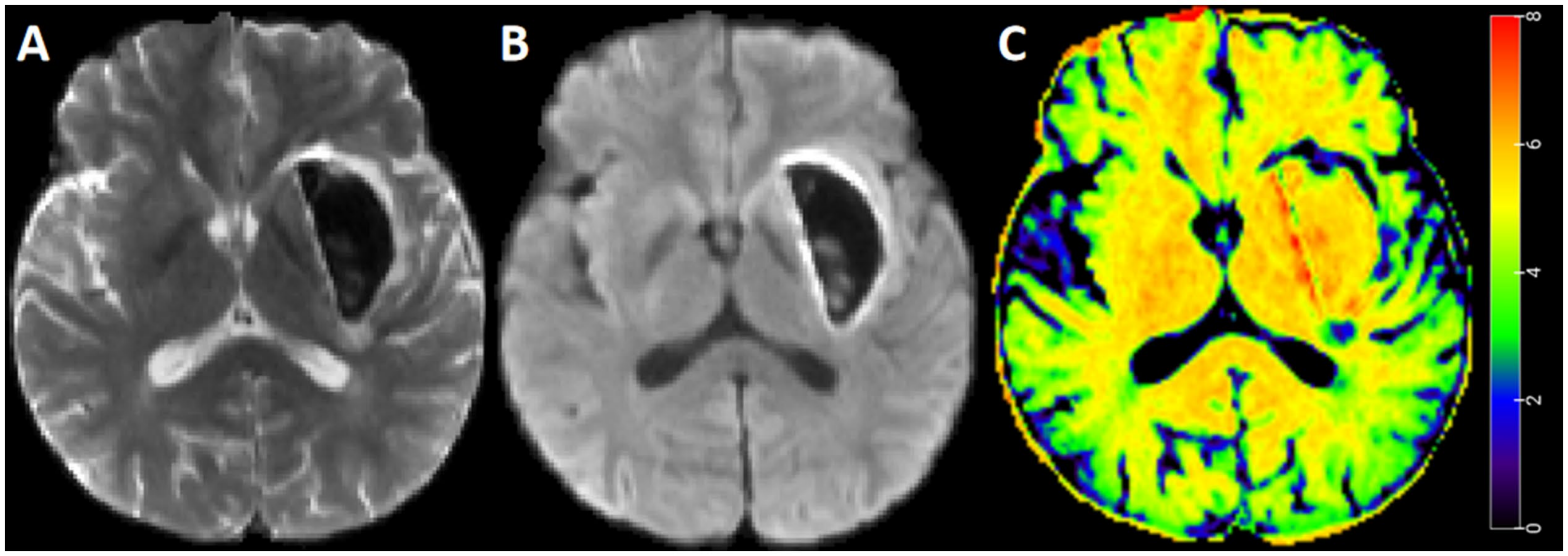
Standard b-value DWI and STI map for representative case. A 45-year-old man with intracerebral hemorrhage. Diffusion weighted imaging with *b* = 0 s/mm^2^
